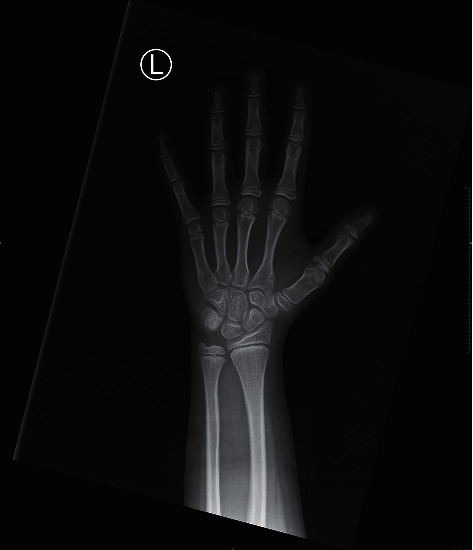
PA radiograph of the left hand for bone age evaluation. A radiograph of the left hand is depicted. The patient's chronological age at the time of the evaluation was 12 years and 2 months. According to the second edition of Greulich and Pyle, the patient's bone age is 11 years. Also noted is a short first metacarpal bone. The remaining bones have normal morphology. There is normal bone mineral density.
